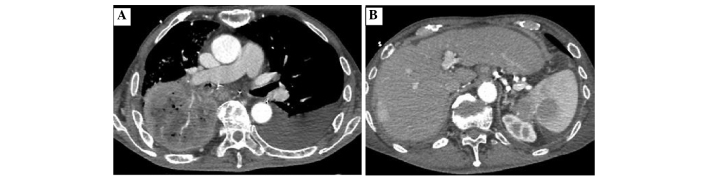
(A and B) Contrast-enhanced computed tomography scan of the chest on third admission showing that the lung mass is larger and is infiltrating the blood vessels. There is also a new solid lesion in the spleen.
